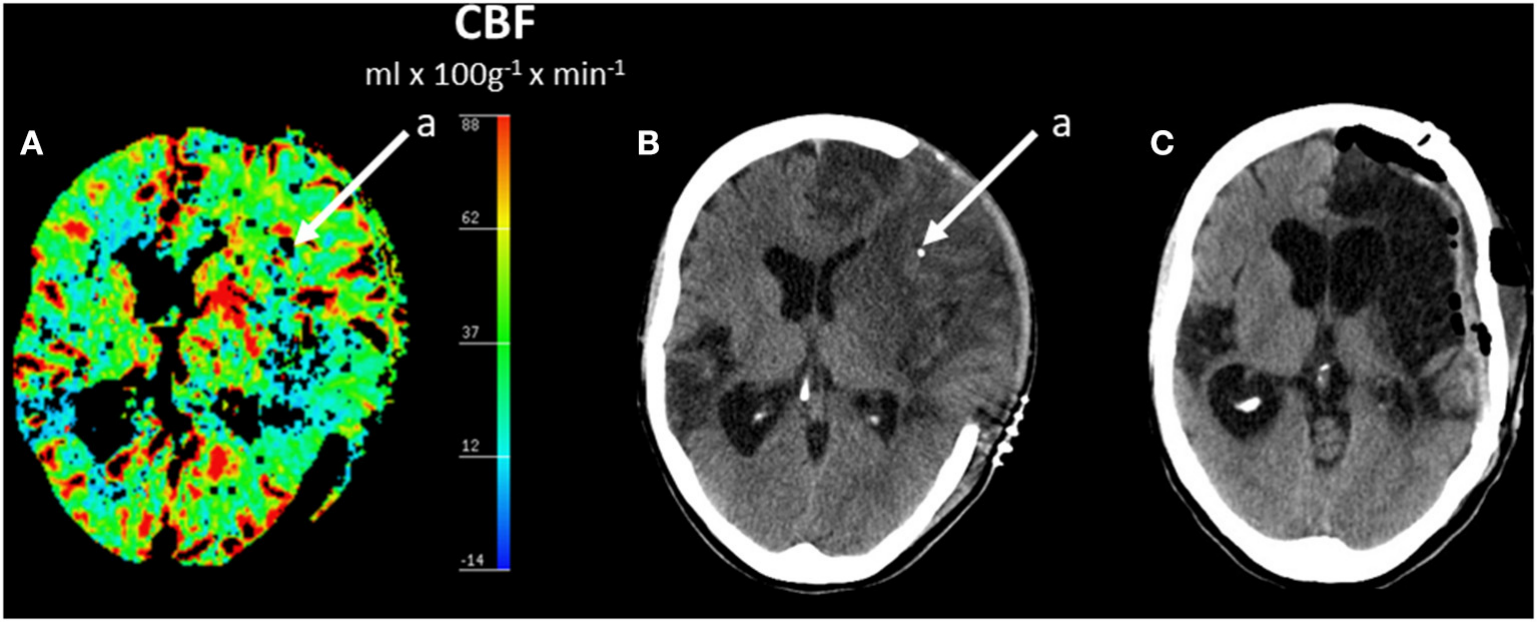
Regional cerebral blood flow (CBF) after hemicraniectomy in a patient with malignant brain edema after middle cerebral artery (MCA) infarct (A) and corresponding acute CT scan (B) and CT scan after 6 months (C). (A,B) Shows the position of one intracerebral microdialysis catheter (a) in the infarcted hemisphere. (C) Shows the CT scan 6 months later after replacement of the skull bone. Data from (89).
